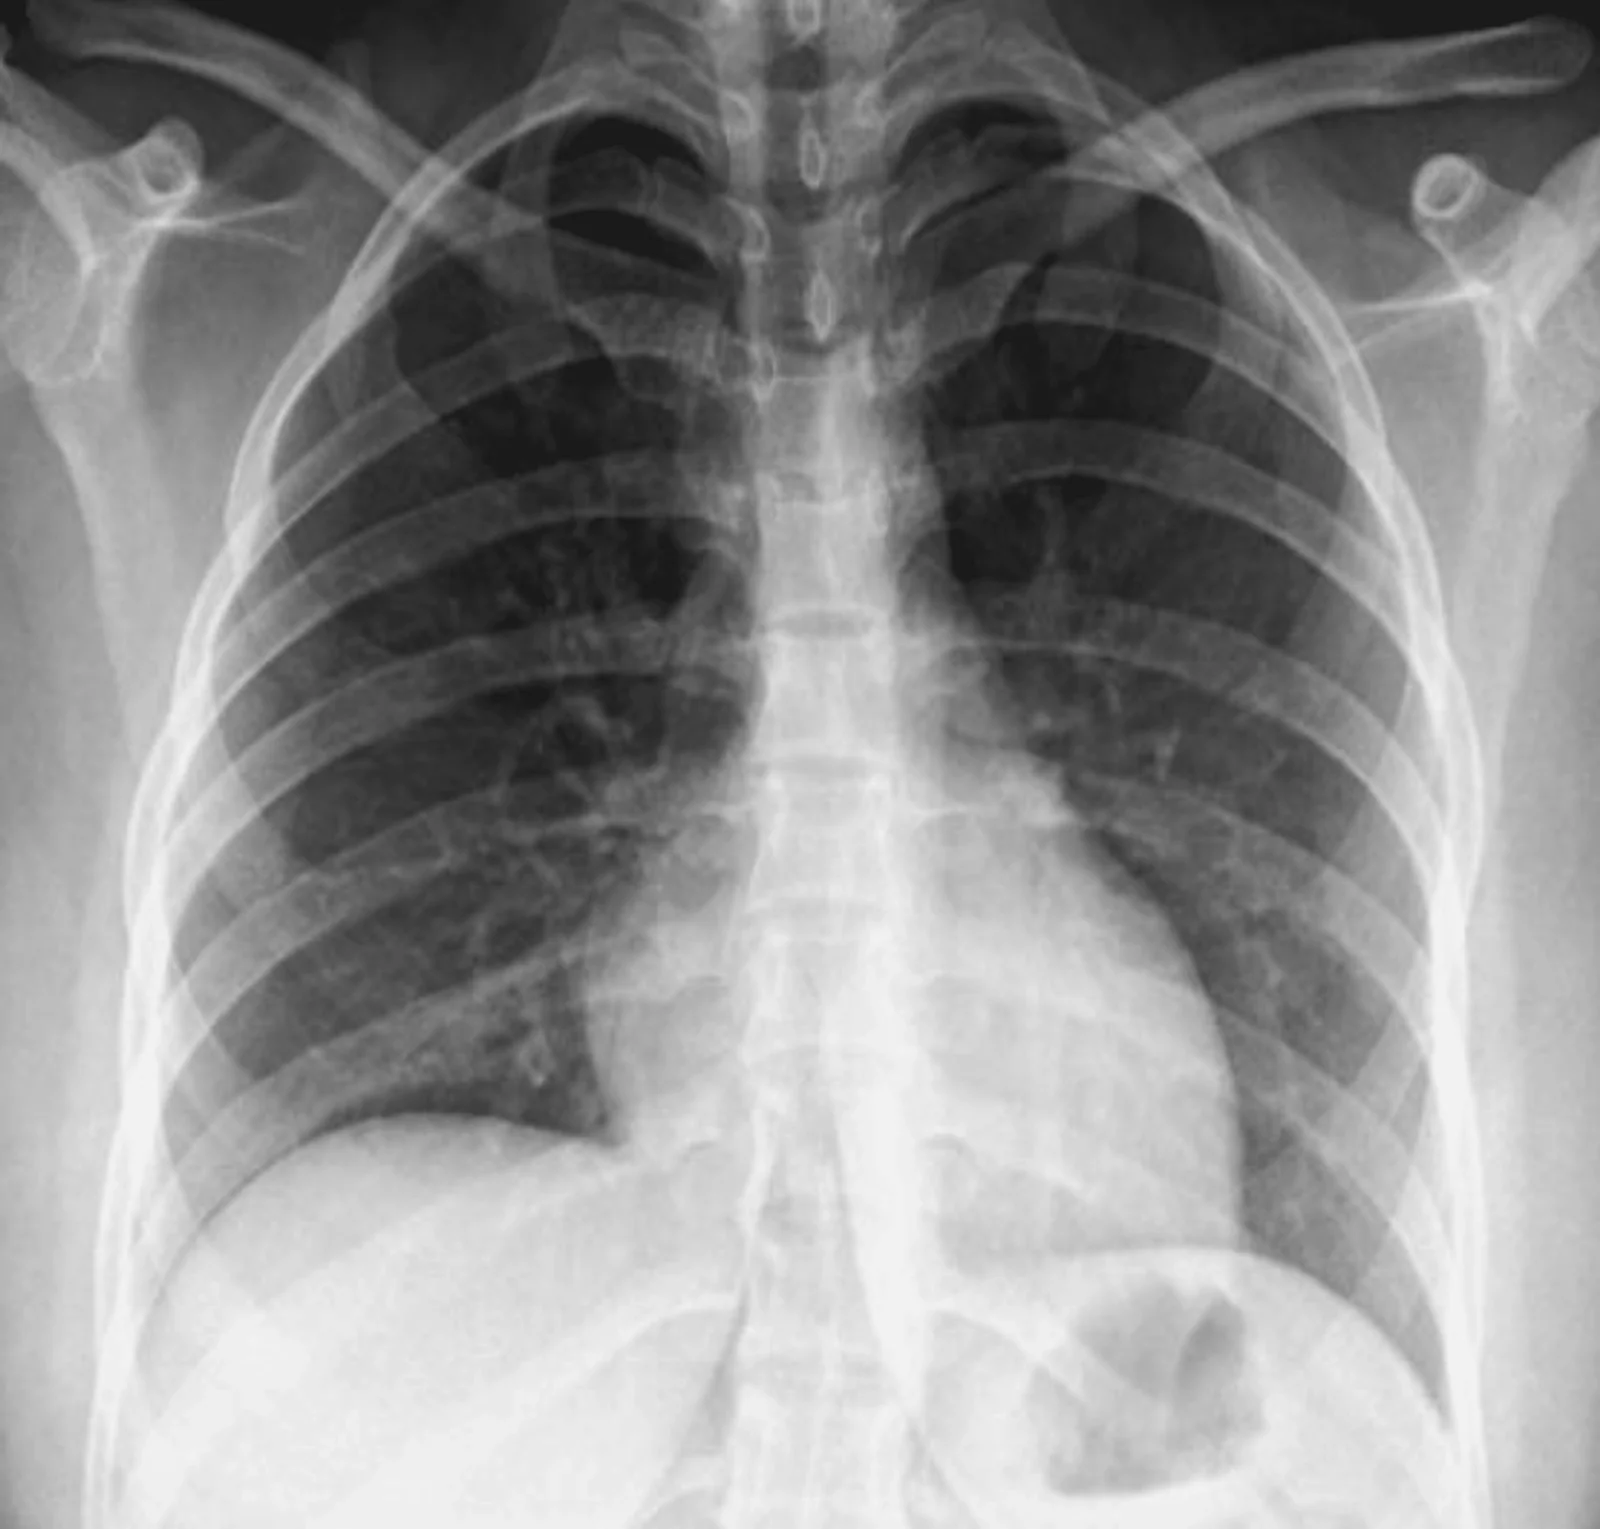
Plain chest radiograph Posterior-anterior, upright, plain radiograph of the chest demonstrating no abnormalities.
